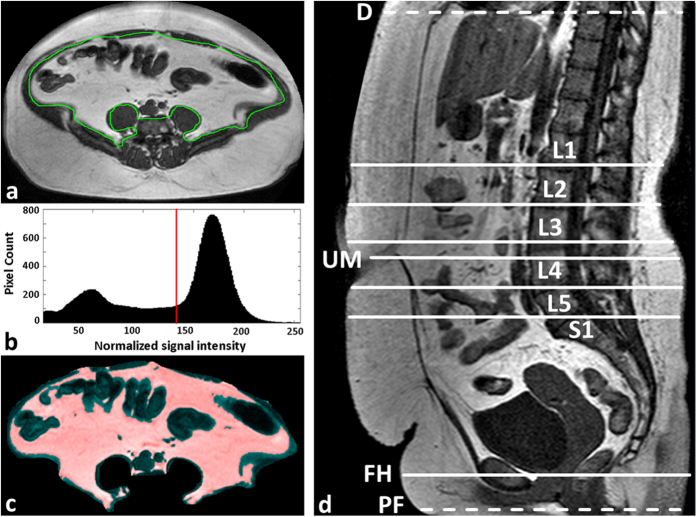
Semi-automated quantification of VAT volume. (a) Automatic segmentation and manual correction of the VAT contour (green). (b) Histogram of normalized MRI signal intensities (SI) of all pixels within the VAT ROI. All pixels with SI above the predefined but adjustable threshold (red line, typically between lean and fat peaks) are considered as VAT. (c) MR image of ROI with VAT pixels overlaid semi-transparently in red. (d) Quantitative VAT areas A_VAT_ were assessed on axial MRI slices at the following reference positions (landmarks): umbilicus (UM), femoral heads (FH), and intervertebral discs L1-L2, L2-L3, L3-4, L4-5 and L5-S1. Total VAT volume (V_VAT_) was analysed between pelvic floor (PF) and diaphragm (D).
